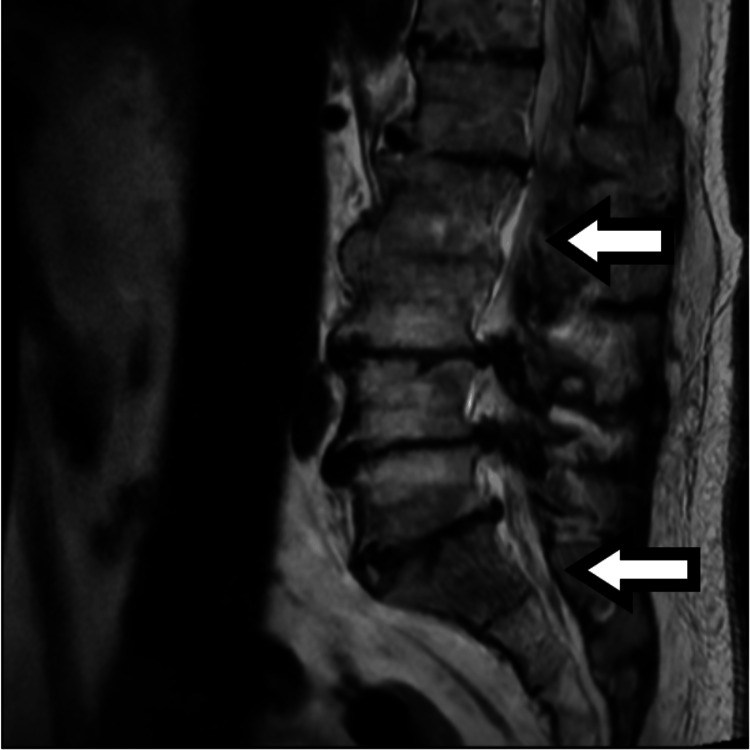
MRI of lumbosacral spine Sagittal view showing acute insufficiency fractures involving S1, S2, and left sacral ala, T1 low and T2 bright signal mass in L3 vertebral body suspicious for a metastatic lesion. MRI: magnetic resonance imaging
